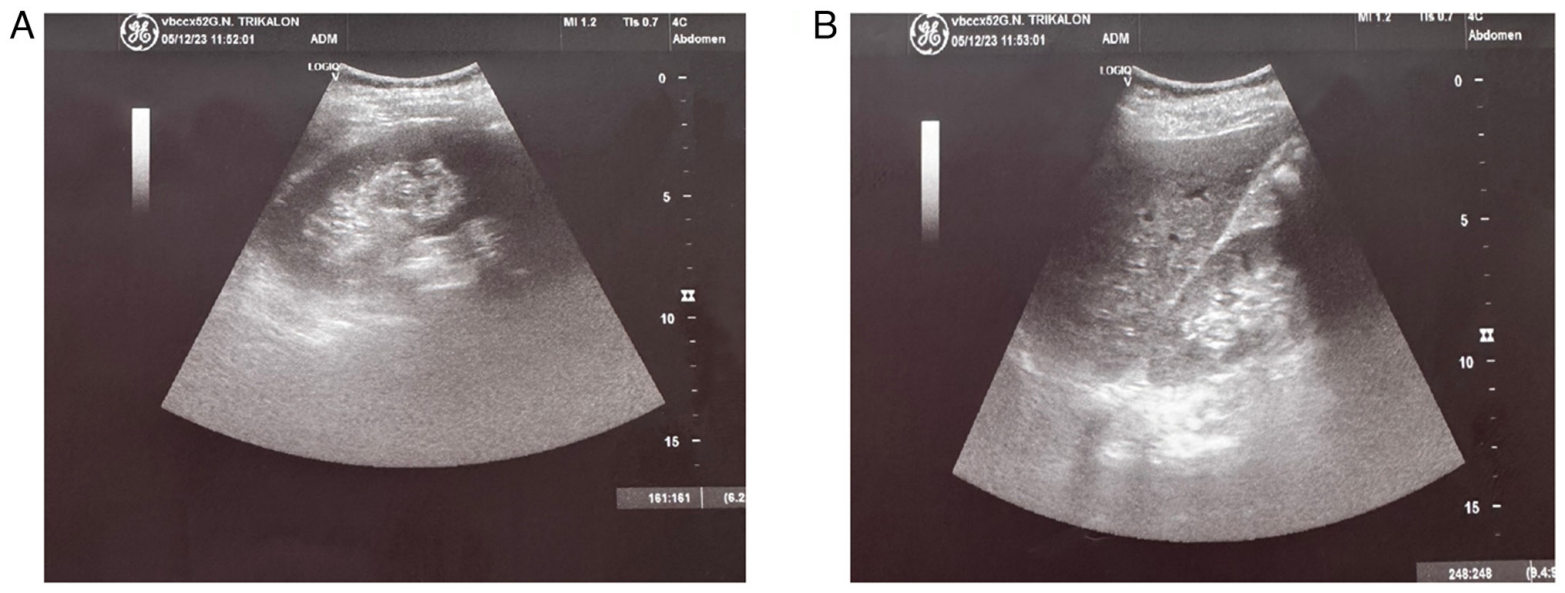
Post-operative ultrasound image of the kidneys, which shows complete recovery of renal morphology and function: (A) Left kidney, (B) right kidney.
